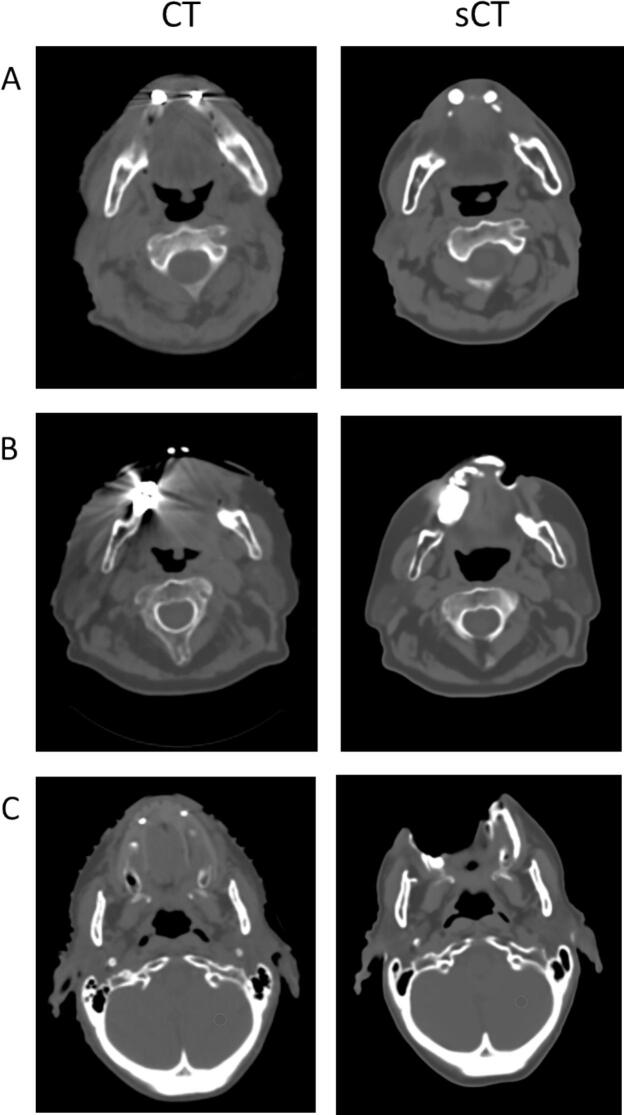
Qualitative examples of metal-induced artifacts in sCT images of patients with dental implants. (A) Case with minimal artifacts, comparable to the reference CT. (B) Case with more extensive distortions affecting the external body contour. (C) Critical case with significant signal loss near the lesion, potentially compromising anatomical accuracy. In our patient cohort, 27% of cases presented minimal artifacts with good overall image quality, 48% showed moderate artifacts with distortions of the external body contour, and in 25% of cases, severe artifacts such as signal voids (holes) in the body contour were observed.
